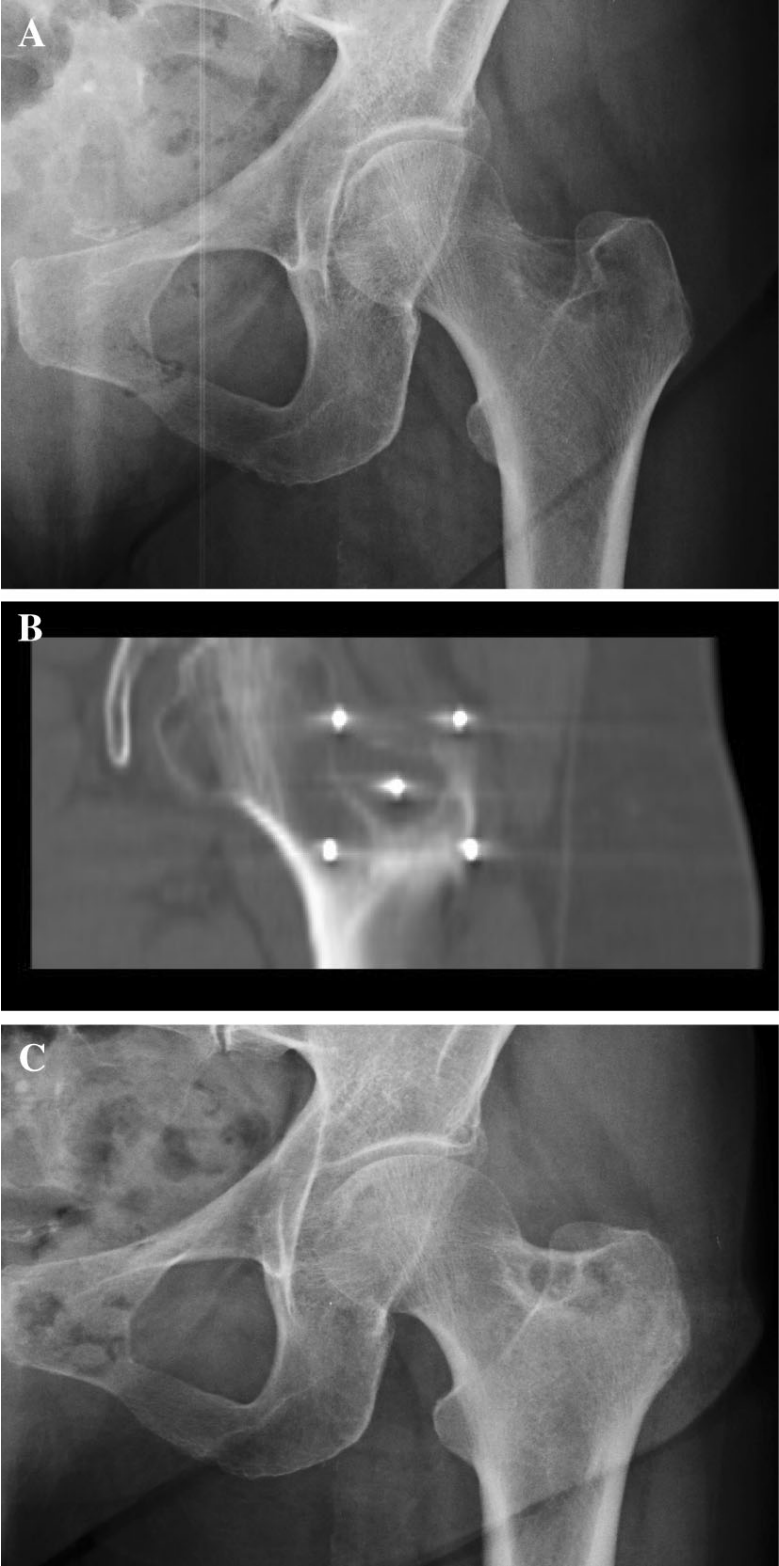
a–c A 69-year-old patient: left femoral neck painful bone metastases, thyroid carcinoma, a CT-guided ECT needles positioning, coronal plane, b X-ray result at 6 months of follow-up; sclerotic rim surrounding the lesion: Partial Response (c)
